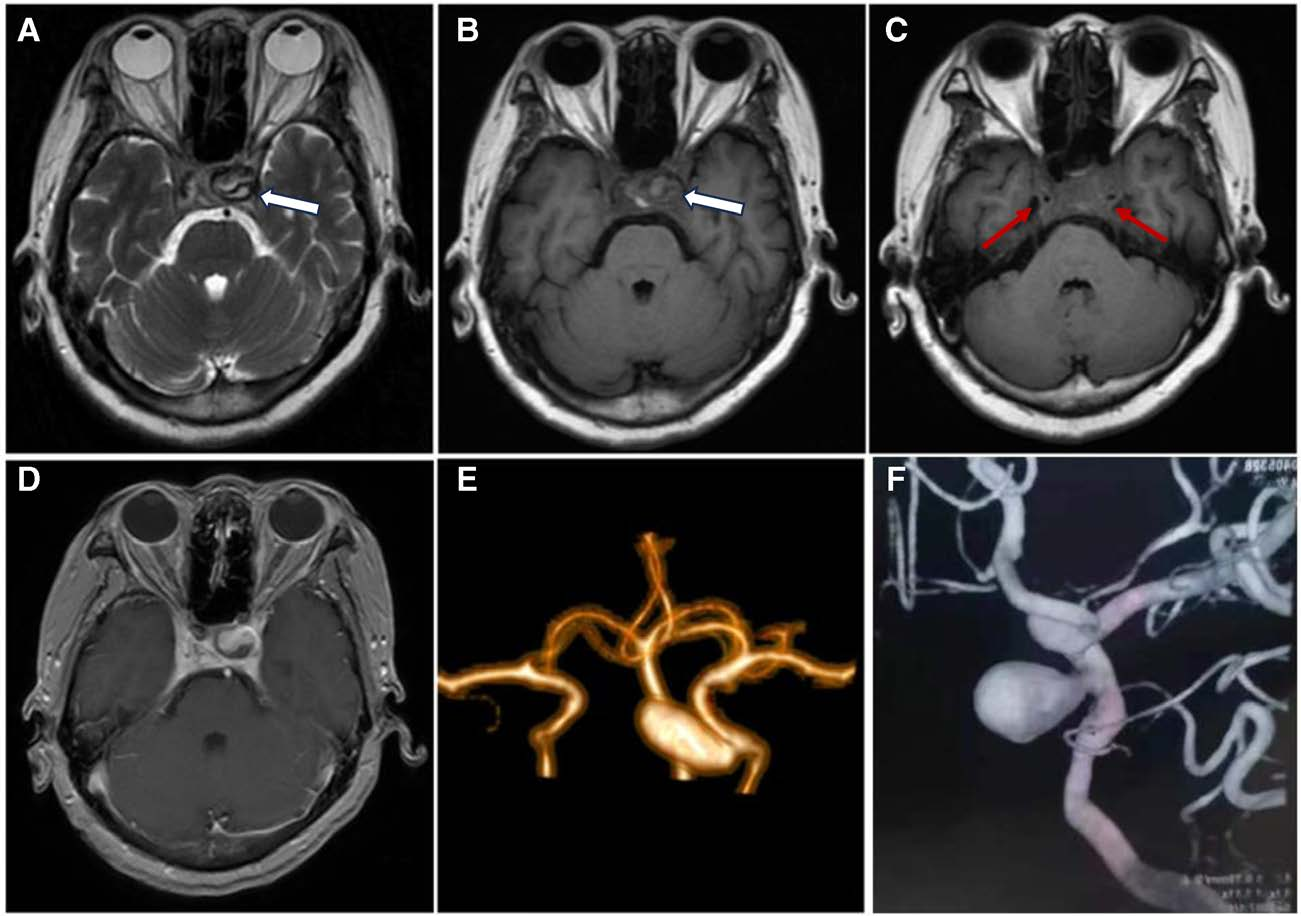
On day 30 after admission, axial T1-weighted and T2-weighted images showed twisty walls of left ICCA (white arrows) and narrow lumens of both ICCAs (red arrows) (A-C). Contrast-enhanced MRI showed the size of bilateral cavernous sinuses shrank (D). CTA confirmed a fusiform aneurysm was located on the left ICCA (E). At 1-year follow-up, left internal carotid angiography demonstrated a slightly widened aneurysm (F). CTA = computed tomography angiography, ICCA = intracavernous carotid artery, MRI = magnetic resonance imaging.
